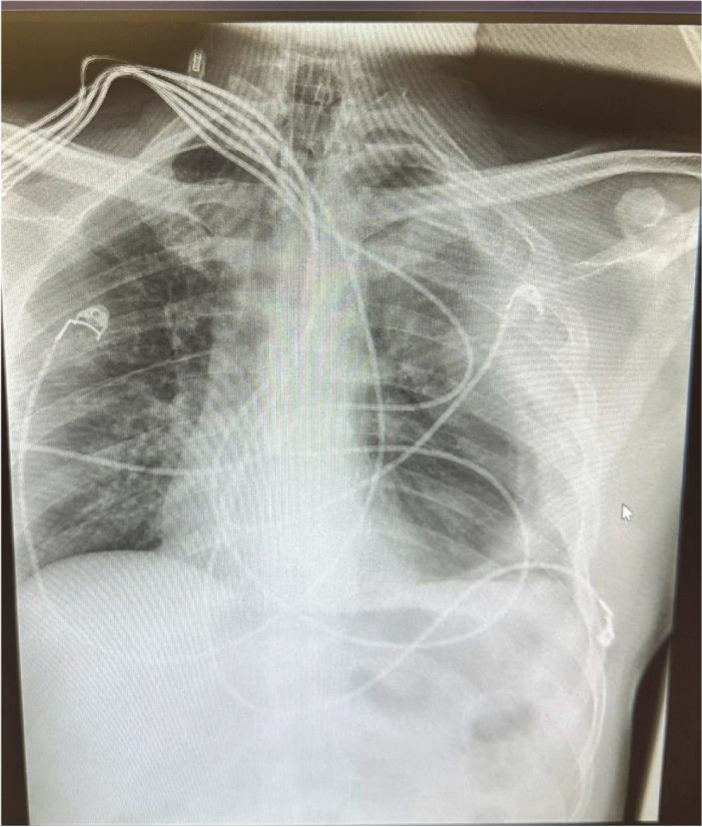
Postoperative chest radiograph on day 2 (frontal view) revealing left sided pleuropneumonia (evidenced by patchy opacities and pleural effusion).
